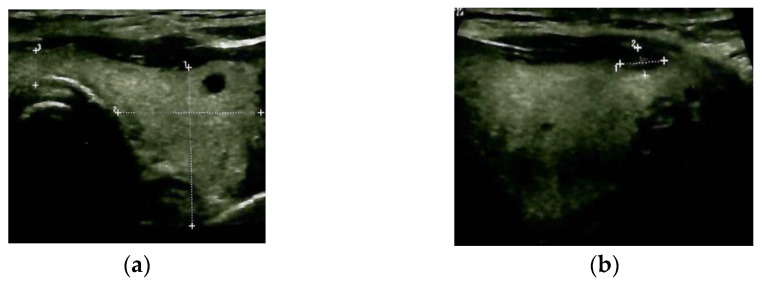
Thyroid ultrasound: multinodular goitre with hypoechoic pattern (a): transverse plane; (b) (right) sagittal plane.
